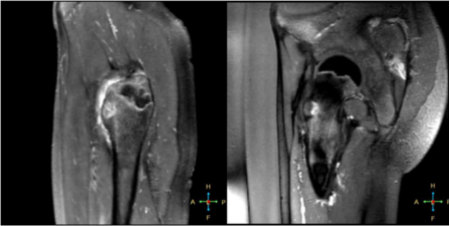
Representative pre and post HIFU sagittal MRI of an osteoid osteoma lesion with gadolinium enhancement. The lesion is fully enhanced before HIFU treatment, and shows non-perfused regions following treatment
